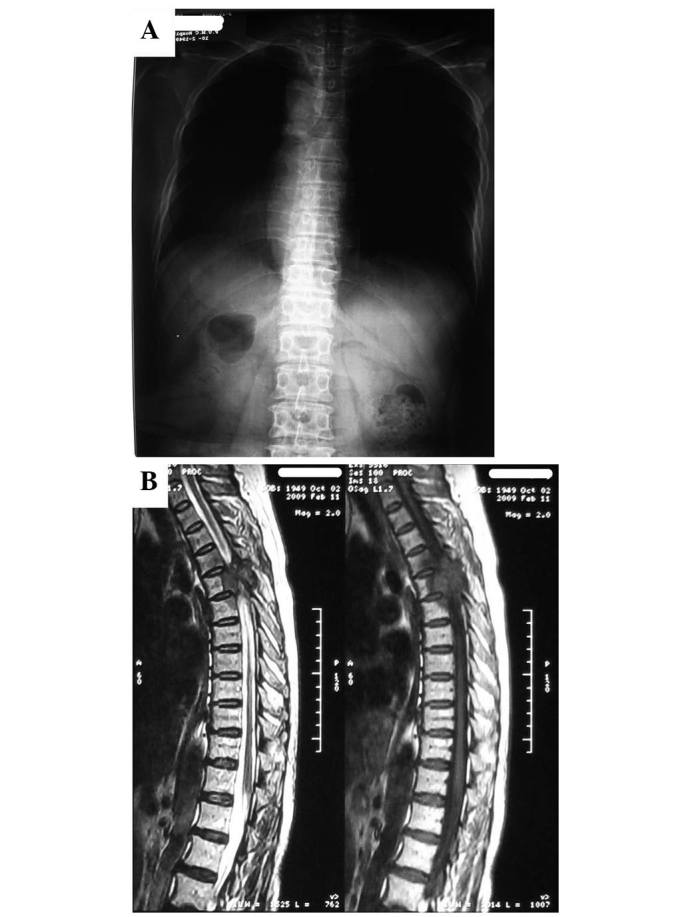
Image examination result of a tumor in the spine. (A) Lateral X-Ray of the thoracic spine of a patient with a thoracic vertebral metastatic tumor (lung cancer), with the lesion located at T4 and T5; (B) magnetic resonance imaging of the thoracic spine showed the vertebral metastatic tumor.
